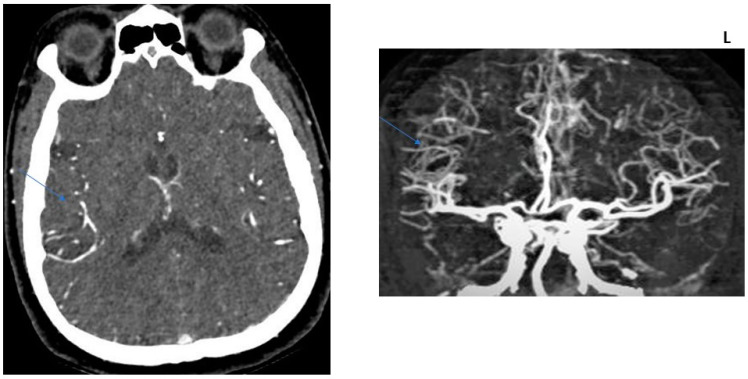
COLLATERAL SCORE: 4–72-year-old female patient. Right MCA infarction. NIHH Score: 12. CT angiography was performed 5.5 h after the acquisition of clinical findings. Left image, “Subtracted MIP image obtained from arterial phase images”. Right image, “Raw image in the axial plane obtained in the arterial phase after contrast injection”. Arrows represent collateral score area.
